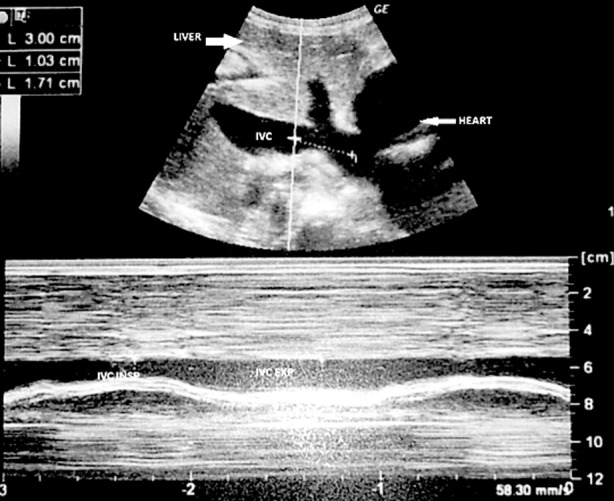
Ultrasonographic determination of inspiratory inferior vena cava (IVCinsp) and expiratory inferior vena cava (IVCexp).
